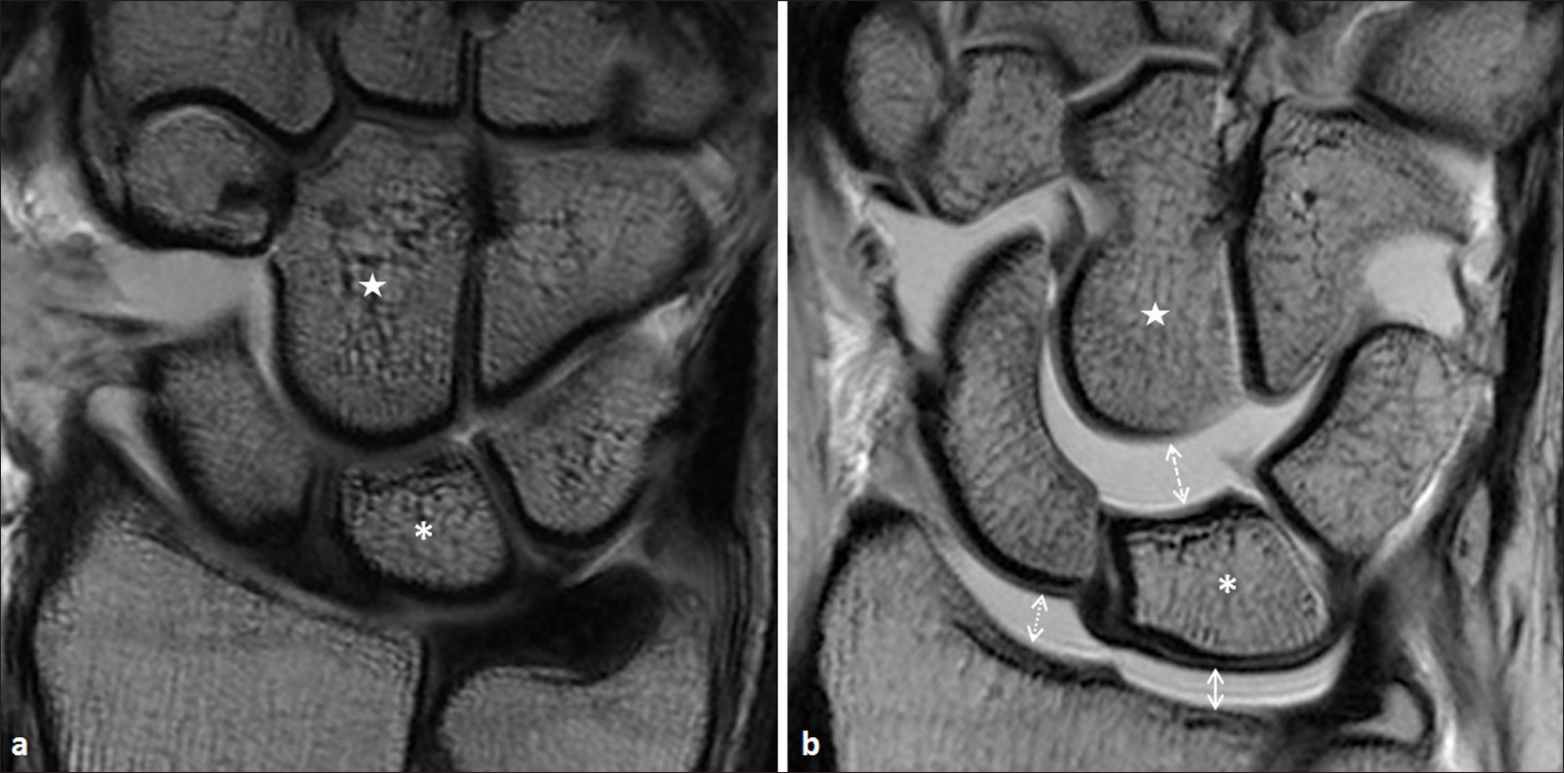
T1-weighted image in the coronal plane in two asymptomatic patients, after intra-articular injection of Gadolinium. Lunate bone is marked with an asterisk, capitatum with a star. a. Without traction. b. During axial traction, in a wrist well responding to traction. There is a marked widening of the radiolunate (*white full double arrow*), radioscaphoid (*white dotted double arrow*) and lunocapitate (*white dashed double arrow*) spaces.
